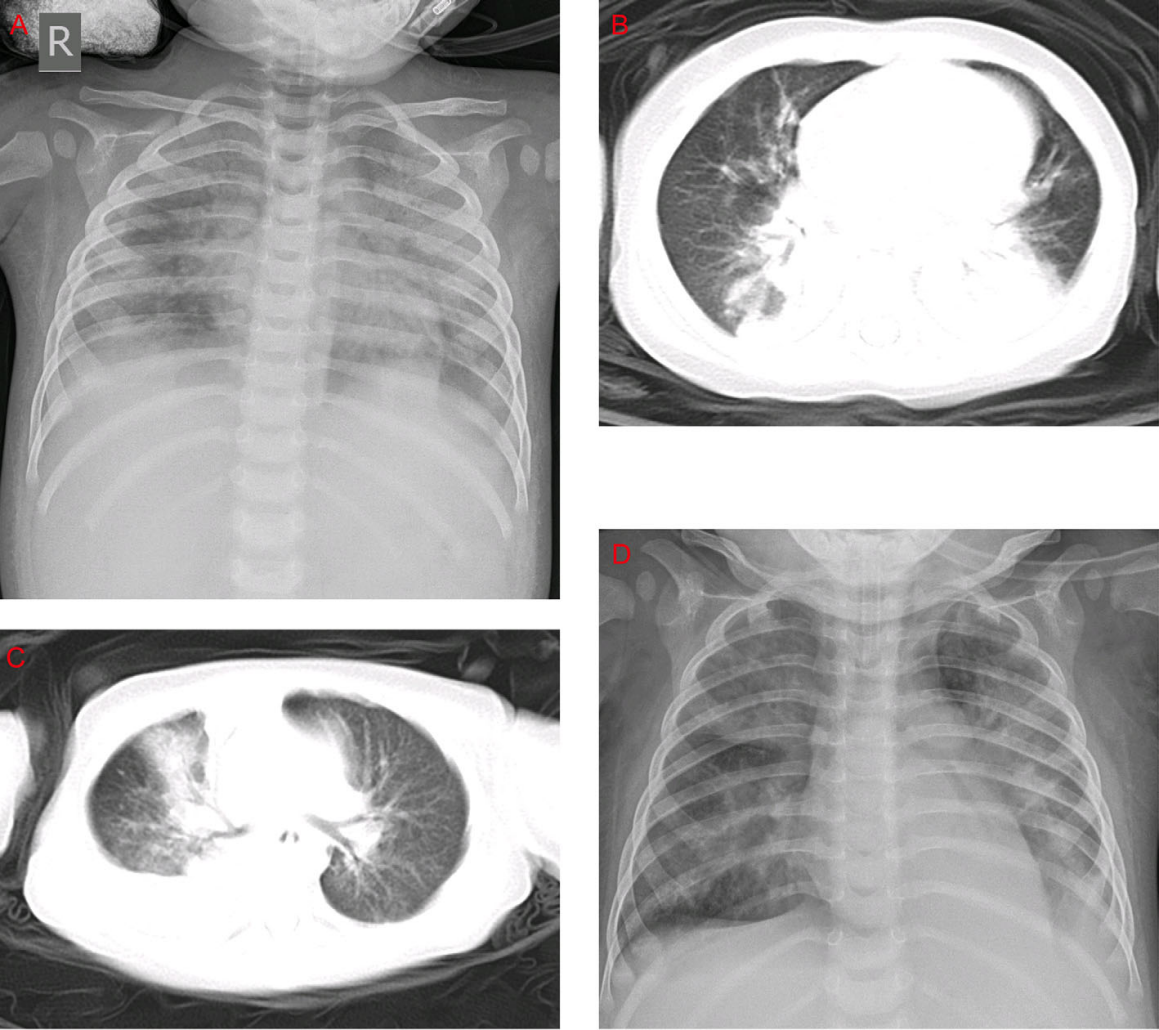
Chest x-rays and chest CTs of patients. (A) Chest x-ray of a 1-year-old boy. The brightness of both lungs was diffusely decreased, showing a large area of patchy shadow with uneven density. Tracheal intubation was seen in the trachea and the heart shadow outline was not cl ear. (B) Chest CT of a 9-month-old boy: mass shadows of high density in both lungs. Bright bronchogram is seen in the lung tissue area of the lesion, which is also called bronchoinflation sign. Condensation shadows were mainly on the lower right lobe. (C) Chest CT of a 11-month-old girl. Condensation shadows were mainly on the right lobe, with right pleural effusion. (D) Chest x-ray of a 10-month-old boy. Patchy blurred shadow was seen in both lungs, the left diaphragm and costal phrenic angle disappeared. Tracheal intubation was seen in the trachea and the heart shadow outline was clear.
